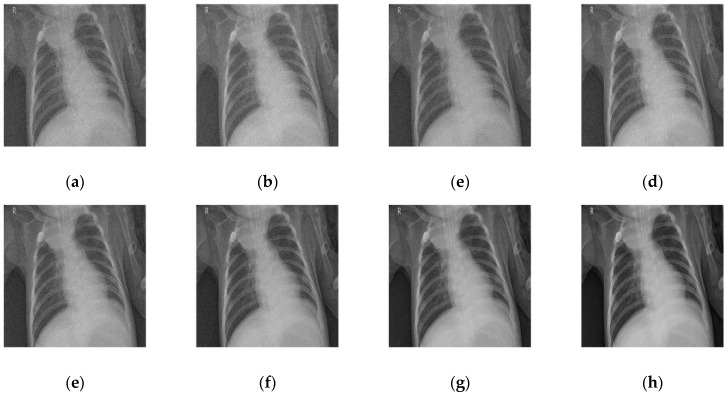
Denoised COVID-19 CT image 2 for the (a) [10], (b) [11], (c) [12], (d) [13], (e) [14], (f) [11], (g) [16], and (h) proposed frameworks, respectively.
